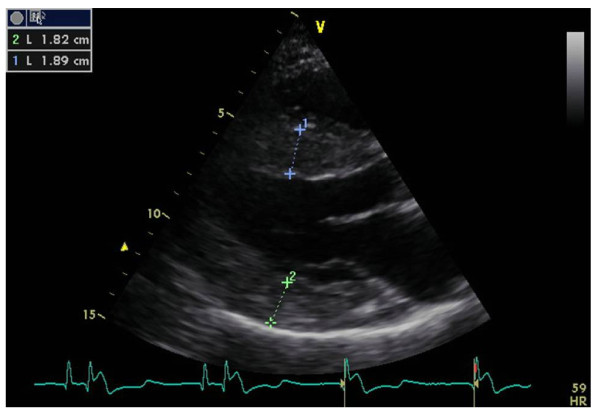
Echocardiography: parasternal long axis showing diffuse left ventricular hypertrophy with increased septal thickness. Courtesy: Pr Albert A. HAGEGE, University René Descartes, Paris, France.
